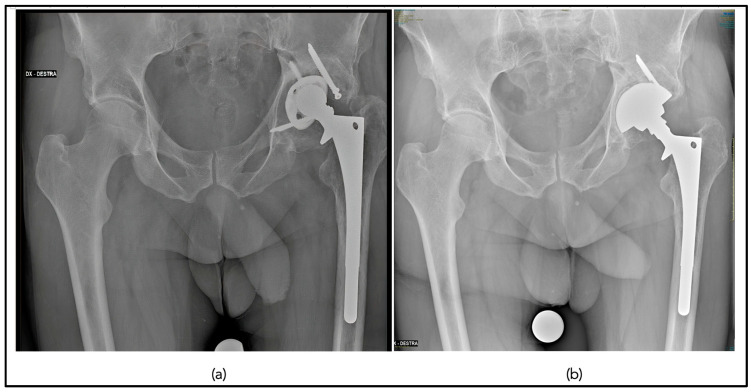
Pre-operative anteroposterior pelvic radiograph demonstrating cup mobilization and polyethylene liner wear (a); post-operative anteroposterior pelvic radiograph at 5 years following stem retention use of the Bioball head–neck adaptor and revision of the acetabular component (b).
